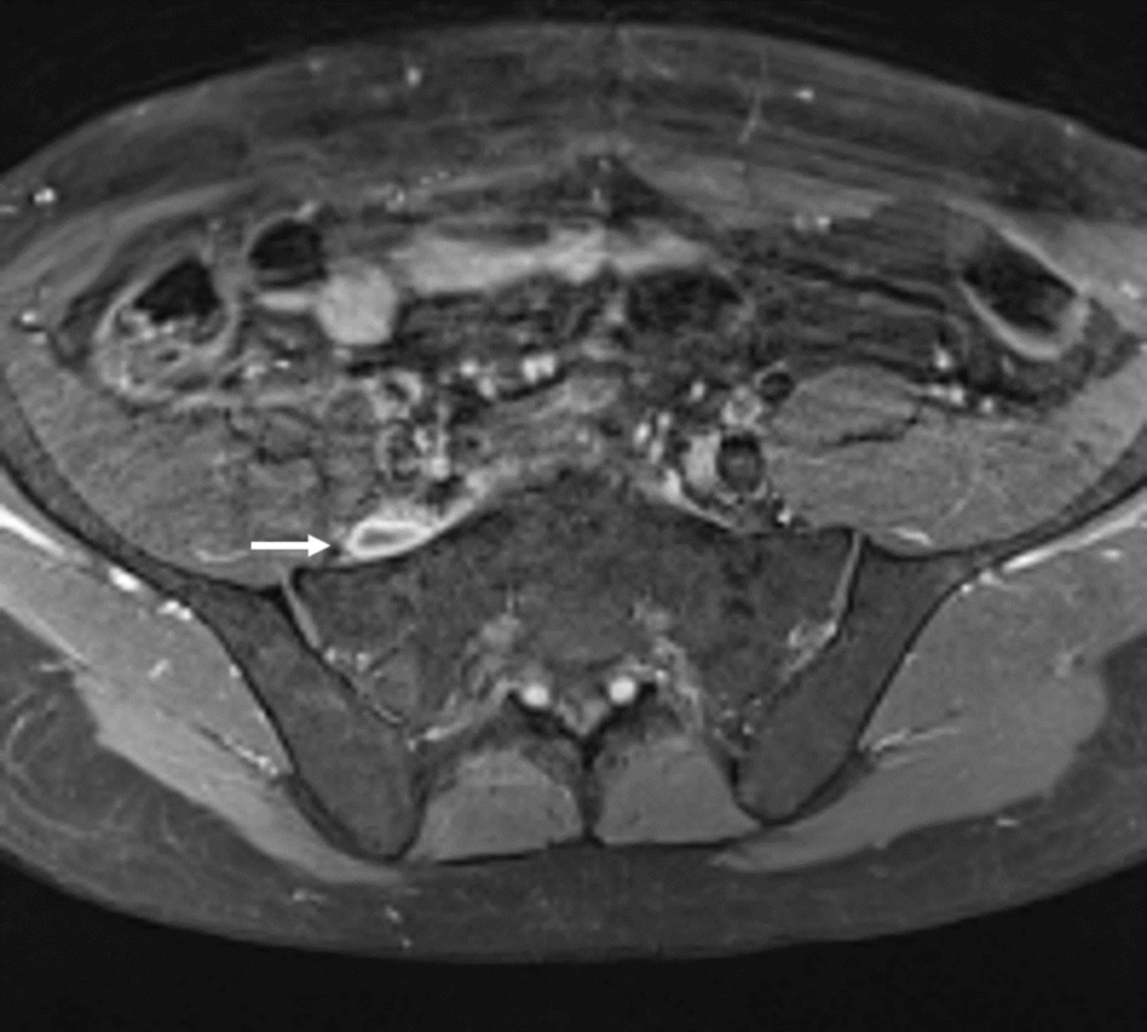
Right pre-sacral mass Axial fat suppressed contrast-enhanced T1 weighted magnetic resonance imaging (MRI) showing peripheral contrast enhancement of the right pre-sacral mass (white arrow)
